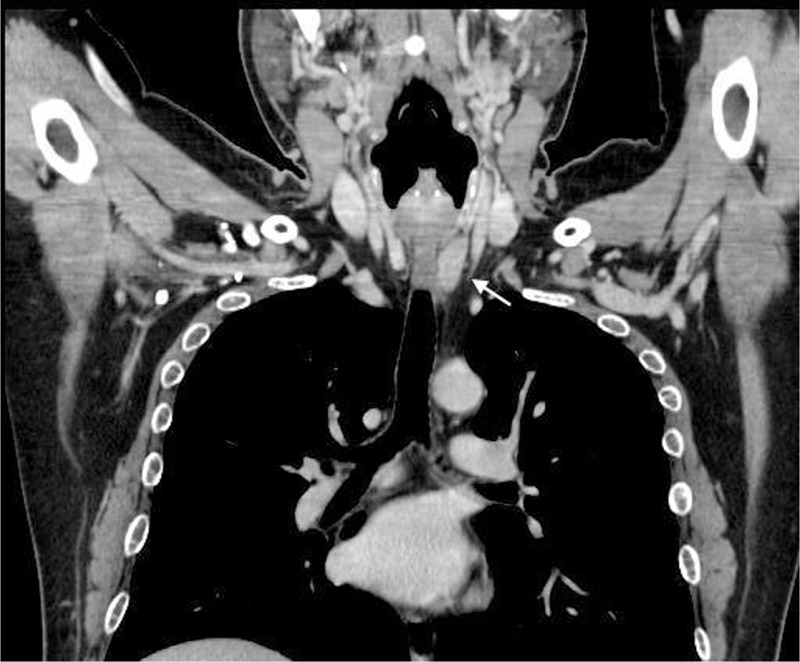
A computed tomography scan showing his parathyroid adenoma (arrow).
